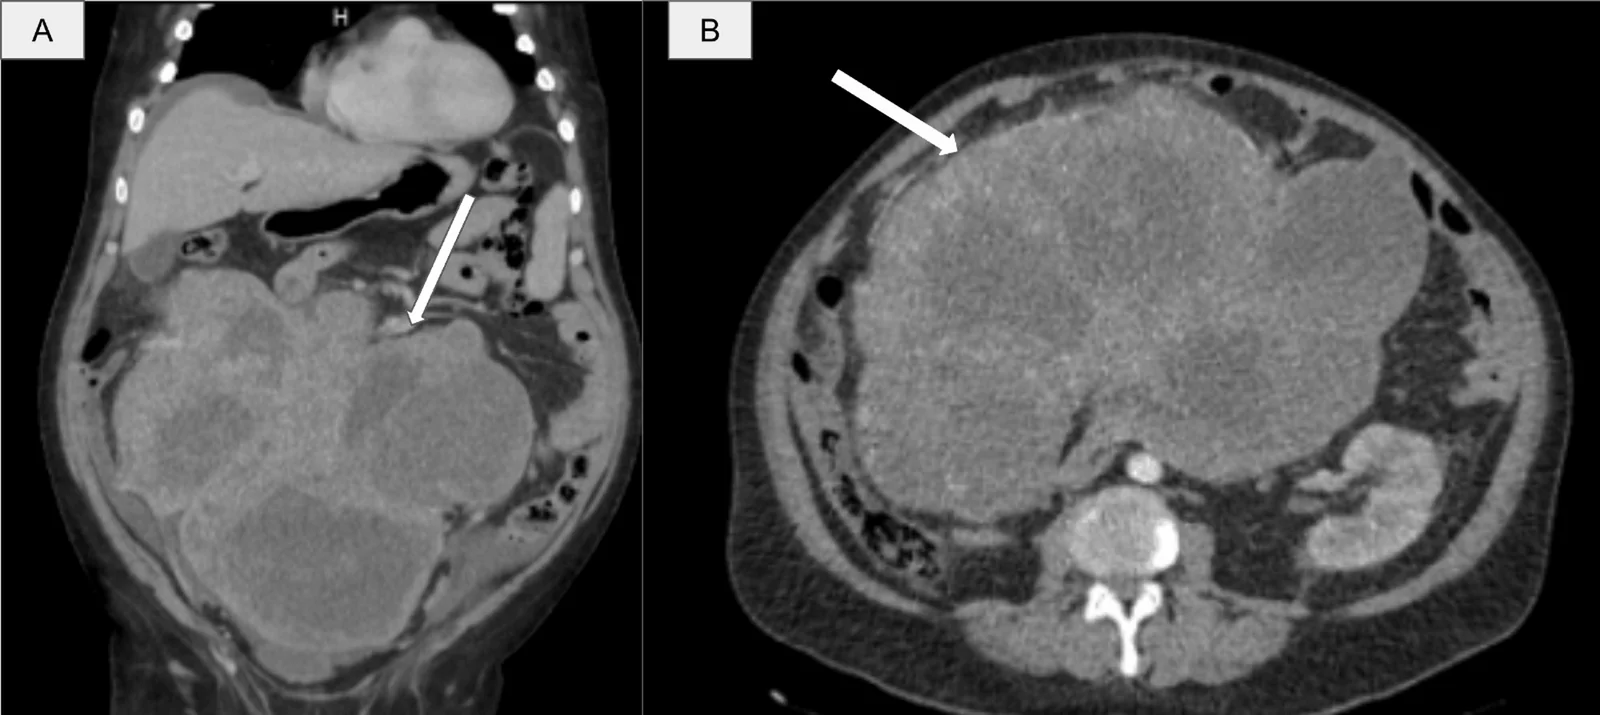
Contrast-enhanced computed tomography (A) Coronal reconstruction showing the cranio-caudal extension of the tumor and near-complete occupation of the abdominal cavity with displacement of surrounding bowel loops. (B) Axial view demonstrating the tumor measuring approximately 29 × 20 cm and producing significant mass effect on adjacent abdominal structures. Arrows indicate the tumor.
